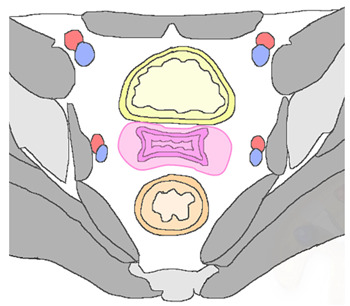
• On T2WI: o In the cervix: a region of intermediate-to-high SI, on a background of low SI change (radiation-induced fibrosis). o In the vagina (vaginal cuff or skip lesions): loss of the linear, low SI of the vaginal vault with an associated intermediate-to-high SI soft-tissue mass. • On post-contrast images: maximum tumor enhancement occurs between 45 and 90 s after contrast administration. • On DWI: an area with hyperintense signal on high-b-value images (with low ADC values on the ADC maps). • On contrast enhanced CT: a soft-tissue mass with early enhancement.
